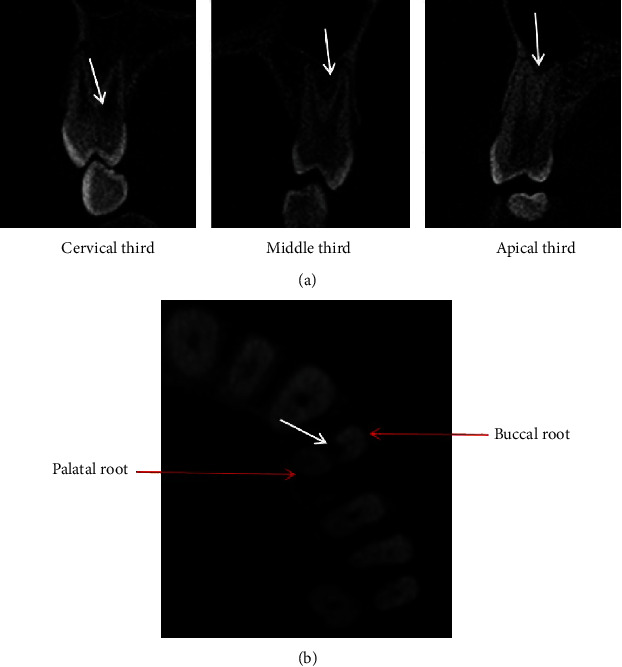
CBCT images showing the level of root bifurcations (a) and furcation groove (white arrow, (b)) in maxillary first premolar. White arrows (a) indicate the positions of coronal third, middle third, and apical third.
